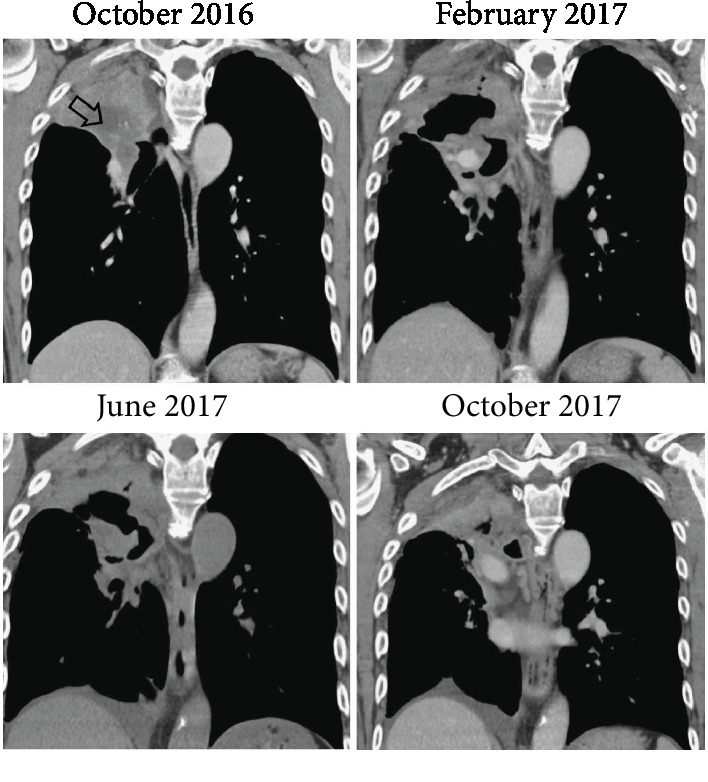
Lung imaging results during the course of the disease. Chest CT imaging is shown for the time between October 2016 and October 2017. For the October 2016 CT image, the lesion is indicated by an arrow.
